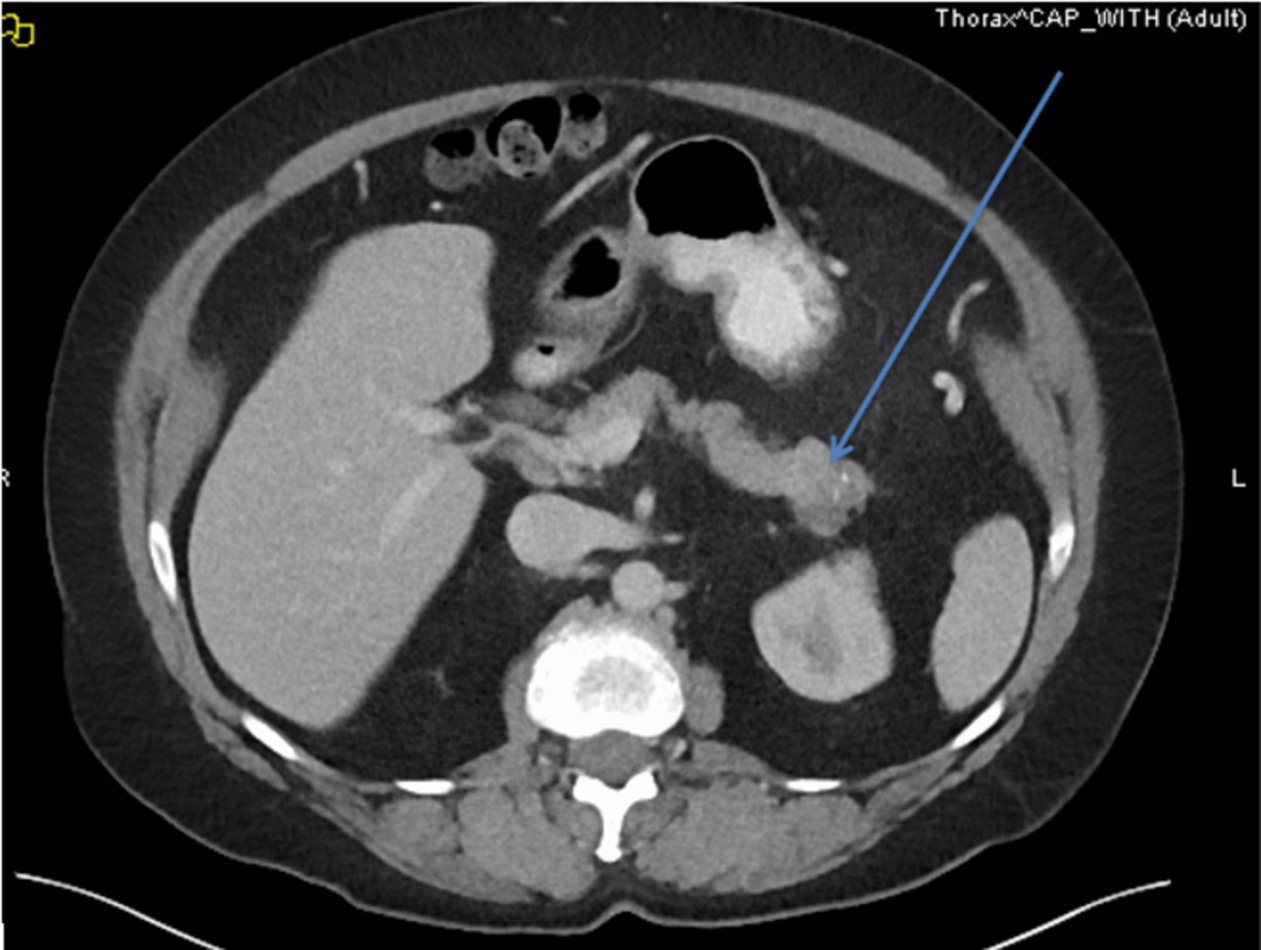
Computed tomography (CT) scan of the abdomen and pelvis with intravenous contrast showing mass in the tail of pancreas
